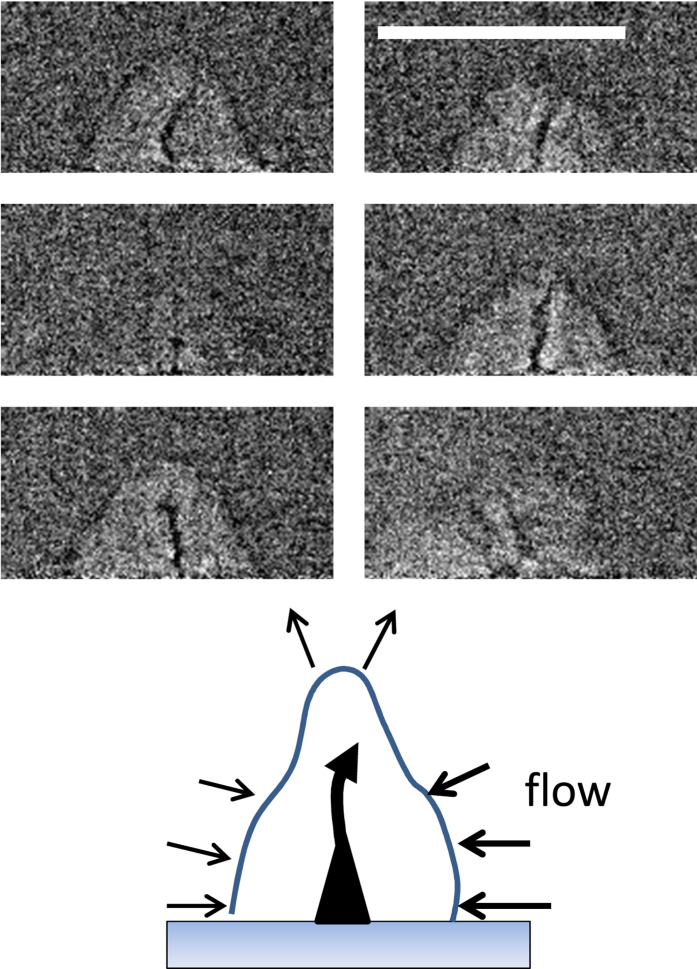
Representative individual radiographs from single laser shots at a fixed delay of ca. 320 *μs* showing the variability of the material ejection. A sketch of the presumptive flow is shown below. The scale bar is 1 mm.
